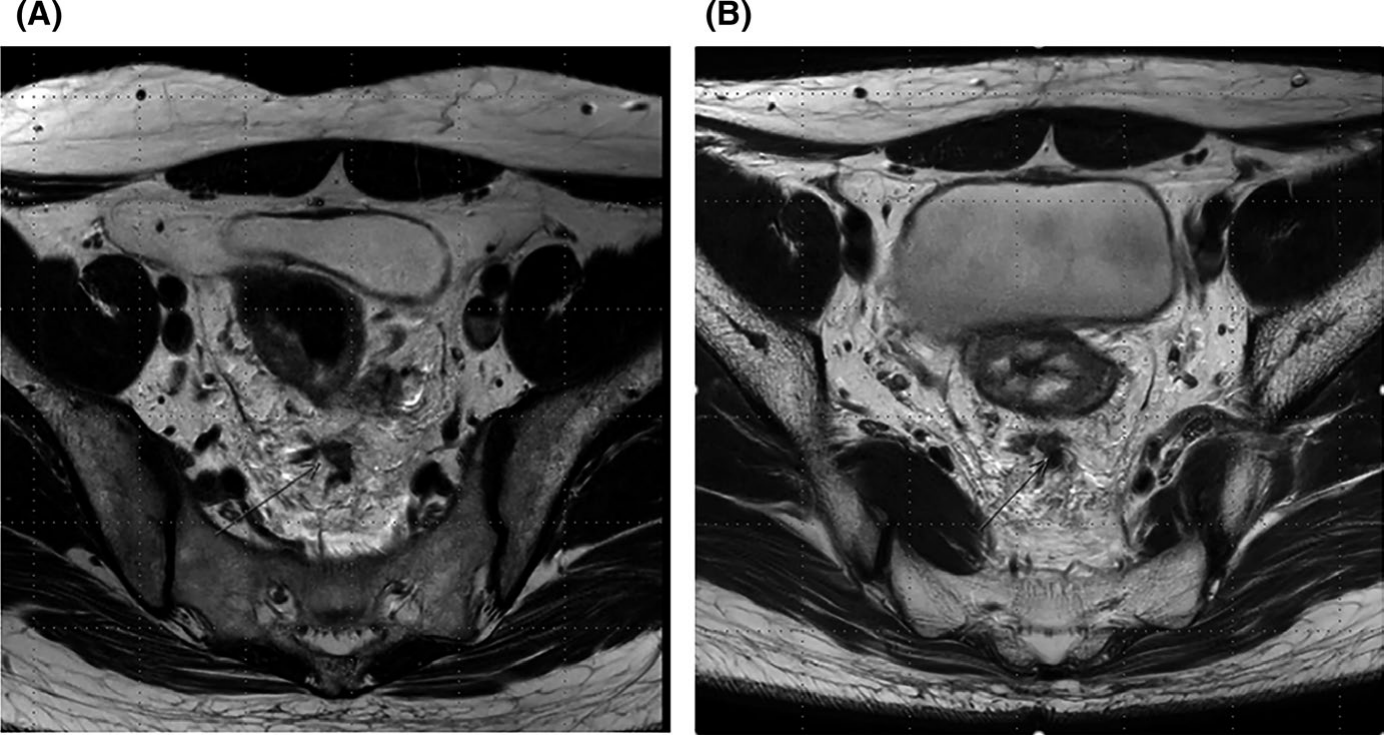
MRI revealing EMVI positivity. A, before neoadjuvant LCRT, B, after neoadjuvant LCRT. EMVI, extramural venous invasion; LCRT, long‐course chemoradiotherapy; MRI, magnetic resonance imaging
